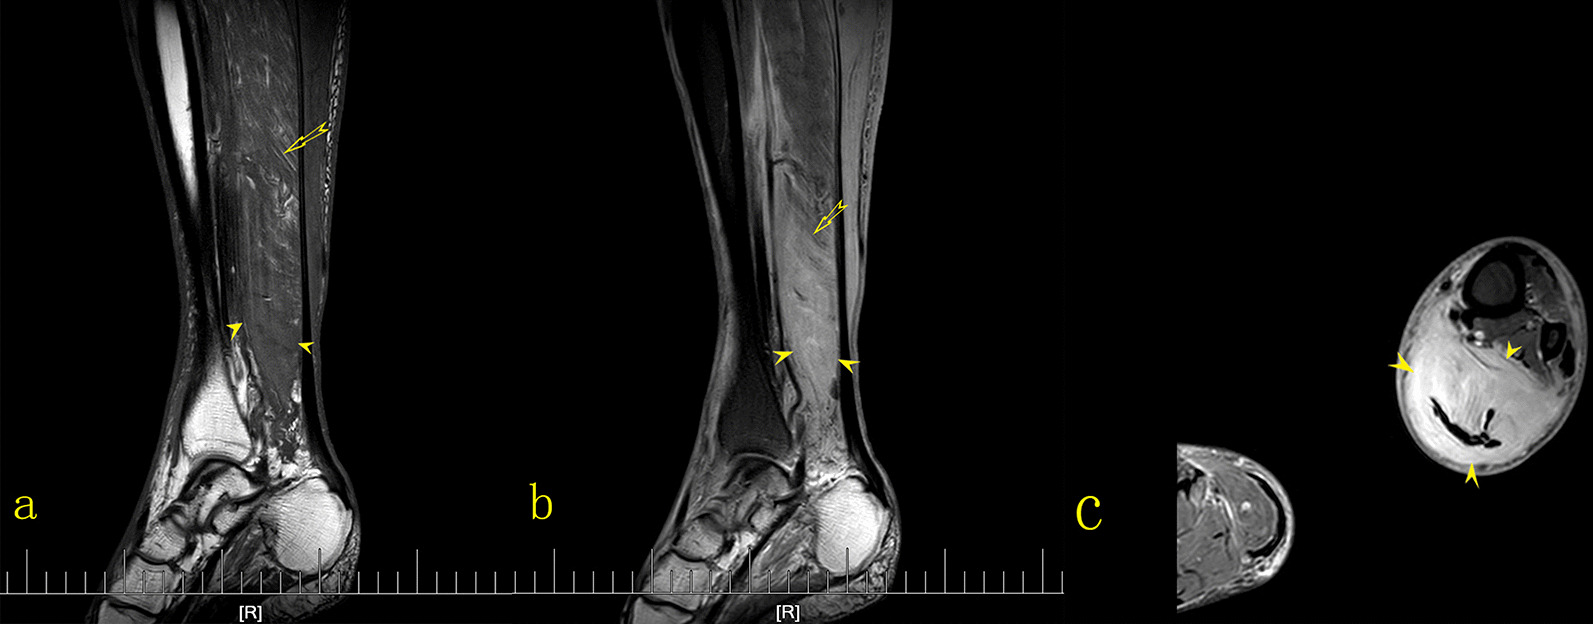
Sagittal T1-weighted images (a), T2-weighted images with fat suppression (b), and axial T2-weighted images with fat suppression (c) showing diffuse swelling that infiltrated the gastrocnemius (*arrow head*), a minimally heterogeneous hypointense signal on T1-weighted images, a hyperintense signal on T2-weighted sequences (*arrow* in a, b), and an ill-defined boundary
